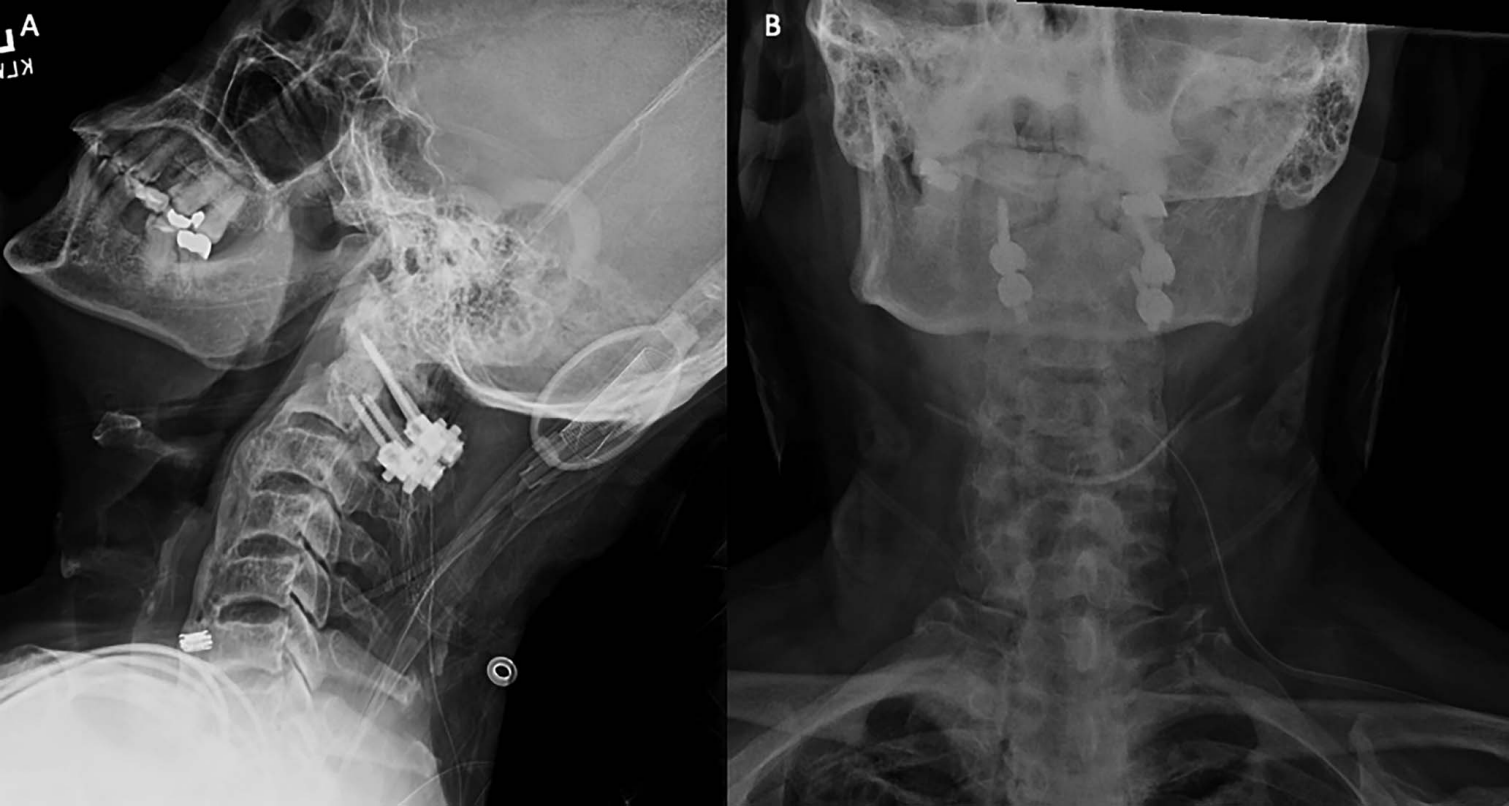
(A) Lateral and (B) anteroposterior plain films of the cervical spine show the initial construct of bilateral lateral mass and pars screws across C1 and C2.
